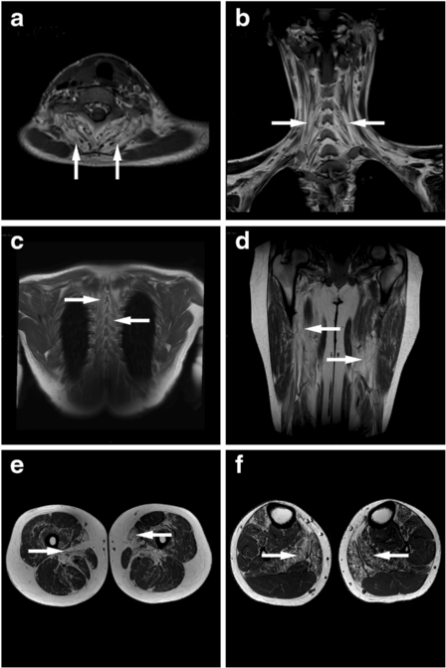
Muscle imaging (MRI) of affected individual 6, family A, 28 year old male. a Axial T1WI of neck demonstrating selective fibro-fatty degeneration of the extensor muscles. b Coronal T1WI of neck showing defuse fibro-fatty degeneration of the extensor muscle extending to the back. c Coronal T1WI of mid back demonstrating defuse fibro-fatty degeneration of the para-spinal muscles. d Coronal T1WI of thigh showing prominent fibro-fatty degeneration of the adductor muscles. e Axial T1WI of thigh demonstrating prominent fibro-fatty degeneration of the adductor muscles. f Axial T1WI of calf showing selective fibro-fatty degeneration of soleus muscle. Arrows highlight loci of fibro-fatty degeneration
